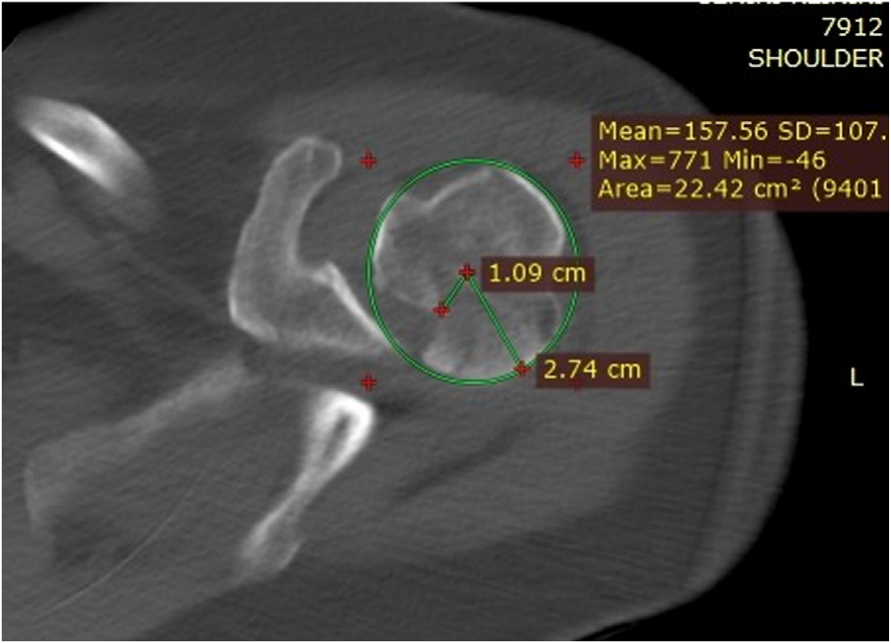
2nd patient: CT scan confirming PSD with associated reverse Hill-Sachs lesion involving approximately 40% of the articular surface of the humeral head.
